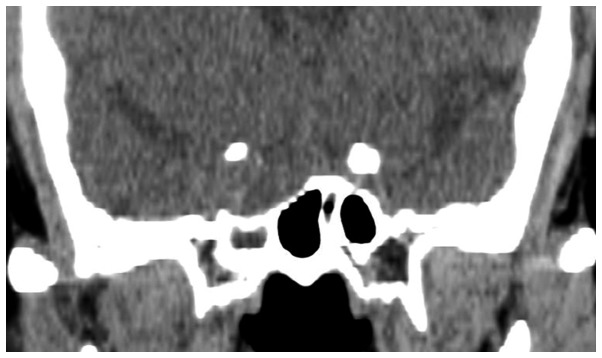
Computed tomography (CT) showing a space-occupying lesion in the sellar area, which affected the right-hand side. The sellar area is expanded and the end of the saddle has sunk.
